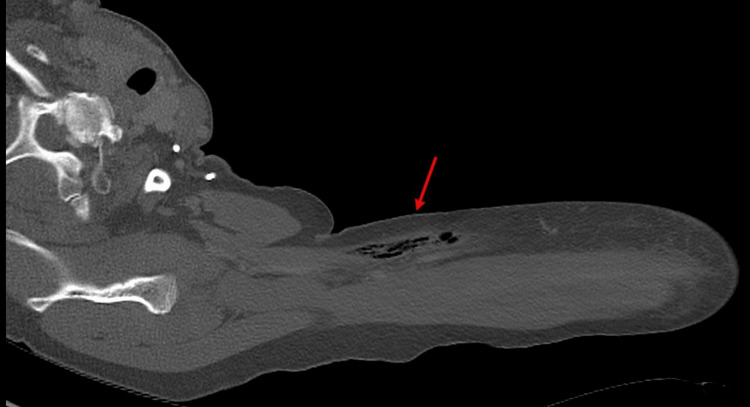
CT scan of left upper extremity showing multifocal regions of intramuscular air tracking along the left medial biceps muscle concerning NF.
